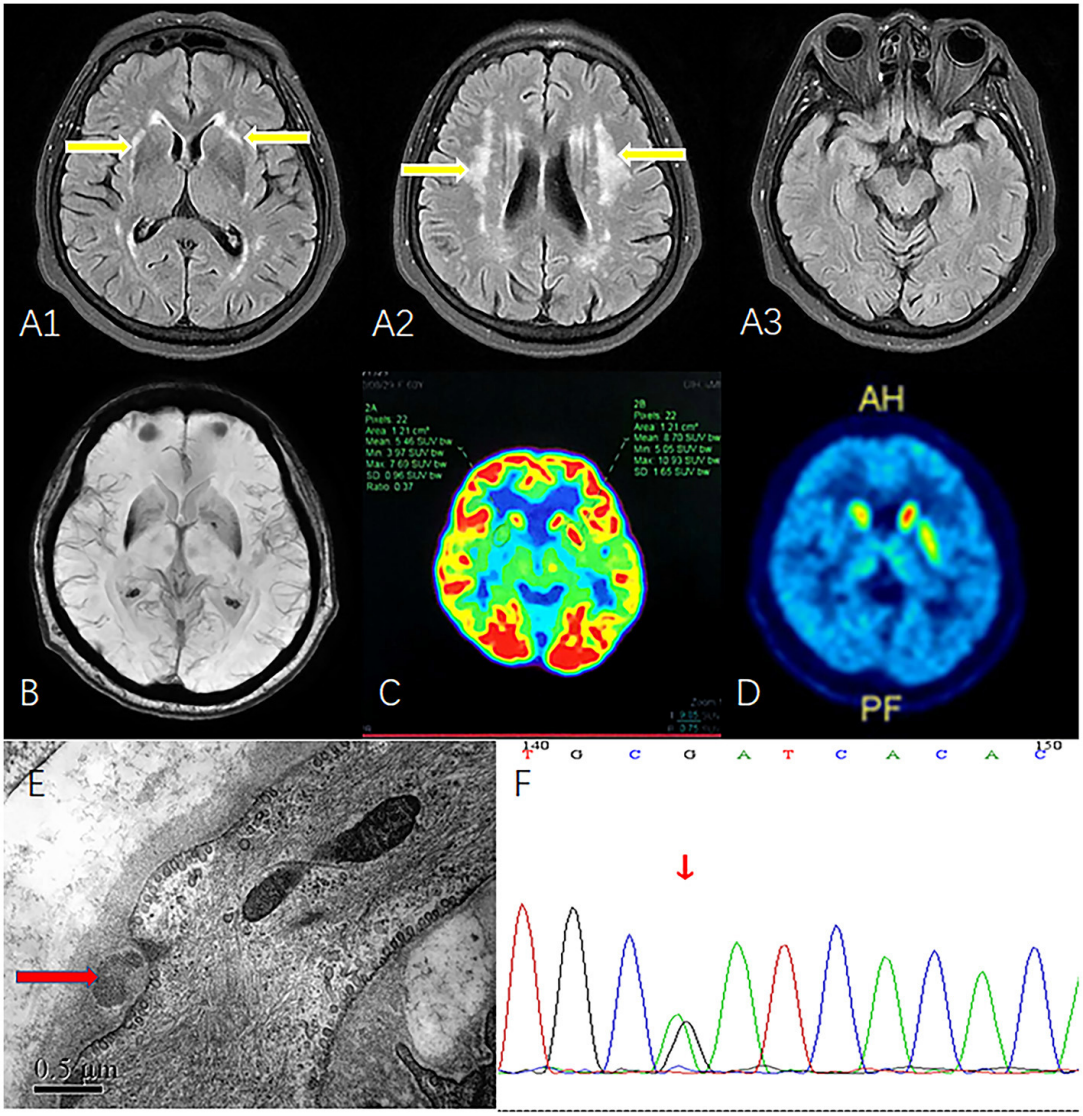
MRI, FDG-PET, VMAT2-PET, skin biopsy, and Sanger sequencing. (A1) FLAIR image shows white matter hyperintensity of the external capsule (yellow arrow). (A2) FLAIR image shows periventricular white matter hyperintensity (yellow arrow). (A3) Bilateral temporal poles show normal signal. (B) Flair image revealed no clearly cerebellar atrophy. (C) FDG-PET reveals hypometabolism in the right putamen. (D) VMAT2 indicates low-intensity signals in right putamen. (E) Skin biopsy shows granular osmiophilic material near the basement membrane of arteriolar smooth muscle cells (red arrow). (F) Sanger sequencing confirmed the mutation c. 1630C>T (p.R544C). MRI, magnetic resonance imaging; FDG-PET, fluorodeoxyglucose-positron emission tomography; FLAIR, fluid-attenuated inversion recovery; VMAT2, vesicular monoamine transporter type 2.
